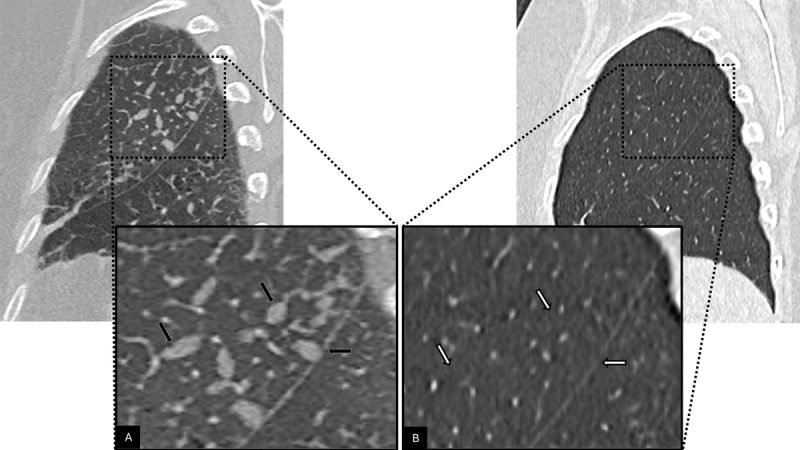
Sagittal CT views at admission (A) and at eight‑week follow‑up (B), with magnified views of selected regions of interest, showing the previously observed ovoid and branched nodules (black arrows) and their resolution over time (white arrows).
